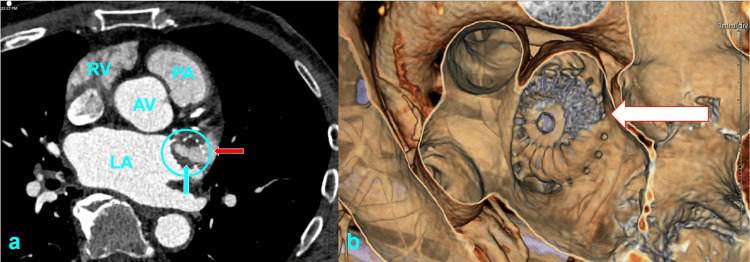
Cardiac computed tomography at 10 months after left atrial appendage (LAA) occlusion. The left panel (a) shows the heart in the transverse plane. Hypodense regions (blue arrow) consistent with thrombus are visualized alongside hyperdense contrast (red arrow) within the LAA occluder (blue circle) and appendage, indicating the presence of a device leak. Right panel (b) is a 3D rendering of the CT, which shows the atrial surface of the LAA occluder device (Watchman FLX) with only partial endothelialization (white arrow). RV: right ventricle, LA: left atrium, AV: aortic valve, PA: pulmonary artery
